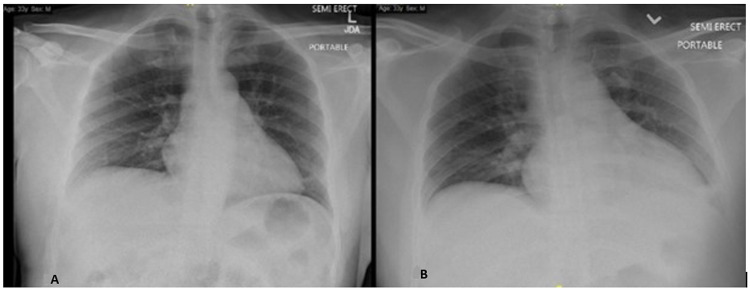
Chest X-ray of the patient (A) chest X-ray showed no evidence of active chest disease; (B) chest x-ray demonstrating cardiomegaly, left basilar infiltrate versus atelectasis
